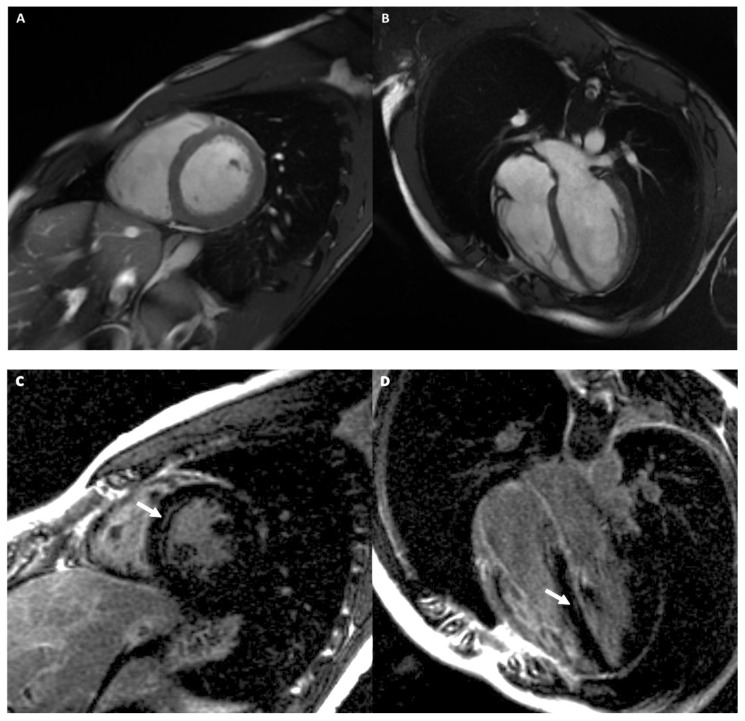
A 40-year-old male athlete (cyclist) with thoracic pain. Cine-CMR images show LV and RV dilation in short-axis (A) and in four-chamber (B) planes. Delay-MRI contrast images demonstrate, in short-axis (C) and in four-chamber (D) planes, linear mesocardial LGE at the mid-basal septum (arrows). The final diagnosis was DCM.
